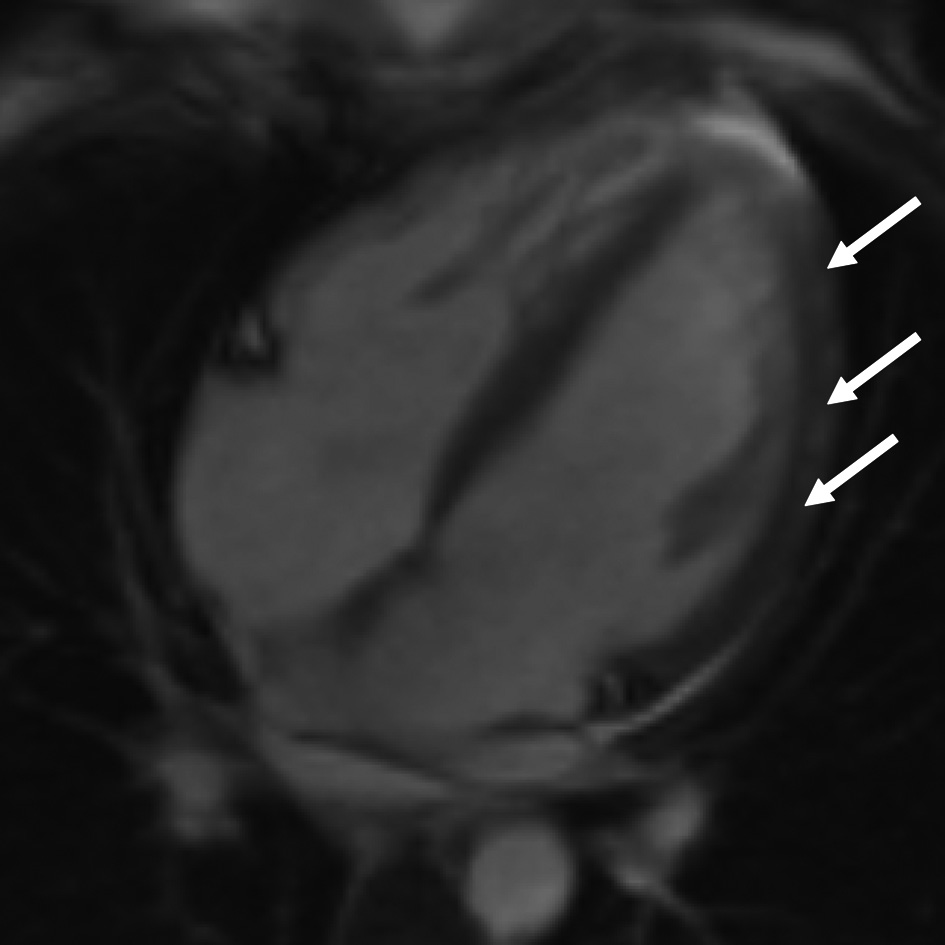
Cardiac MRI. Delayed-enhancement sequences performed after perfusion of gadolinium demonstrated a diffuse lumpy subepicardial enhancement (white arrows).
